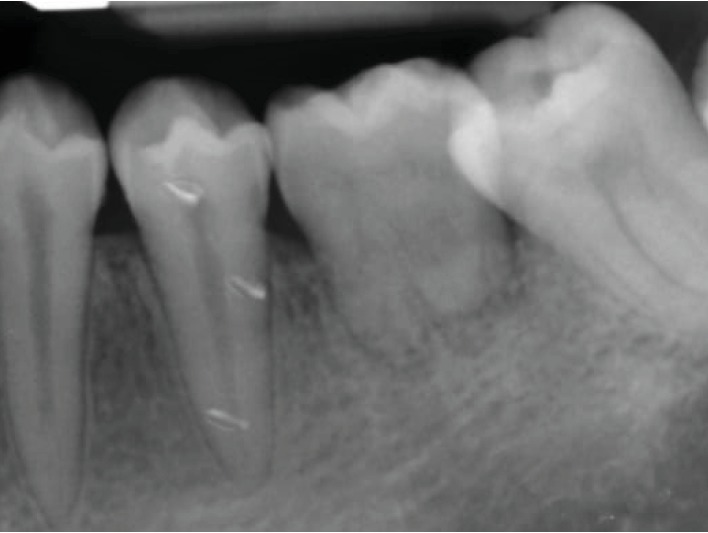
2-year periapical radiograph showing increased root length and open apex.
